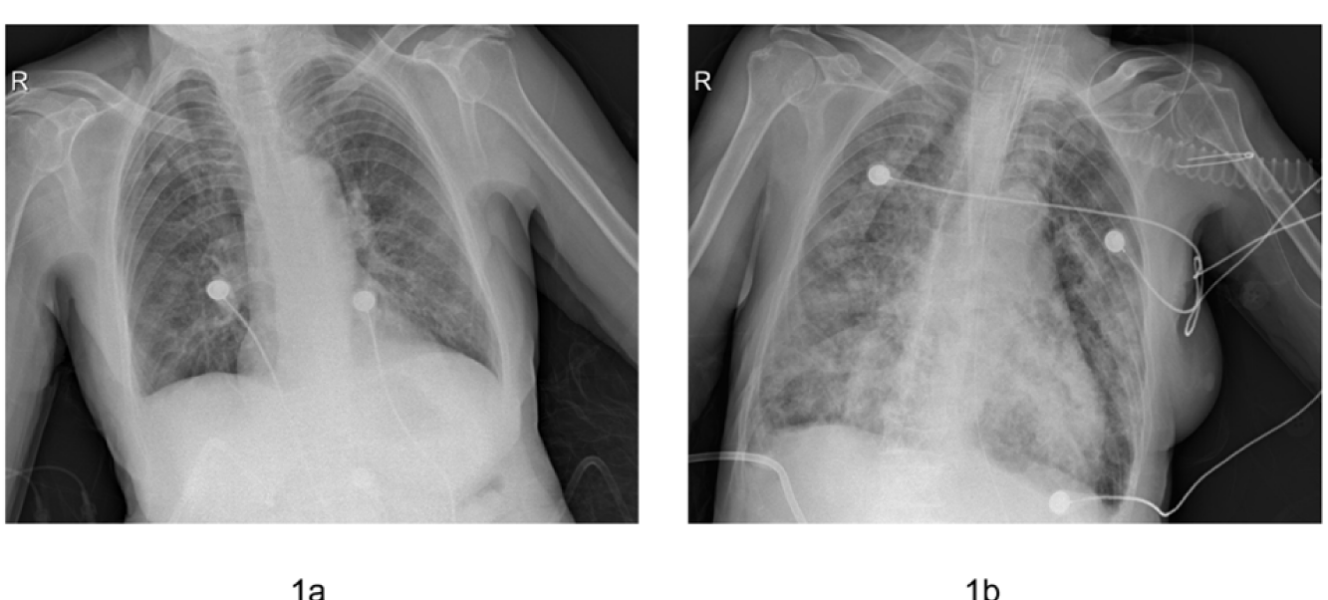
Comparison of the chest X-ray performed upon admission to the hospital (a) and upon intubation (b)
